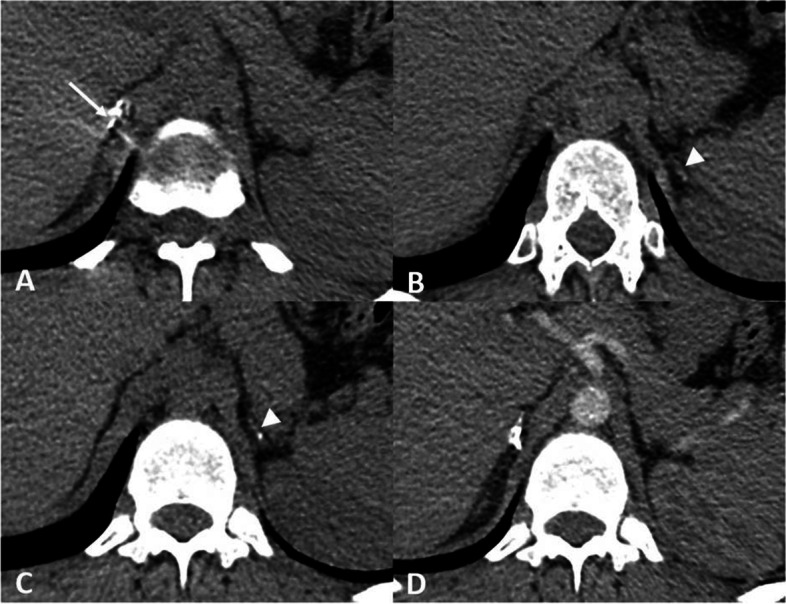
A, B & C abdominal CT scan without contrast (A) right adrenal with coarse calcification (B) & (C) atrophic left adrenal with fine calcification (D) abdominal CT scan with contrast (venous phase)
